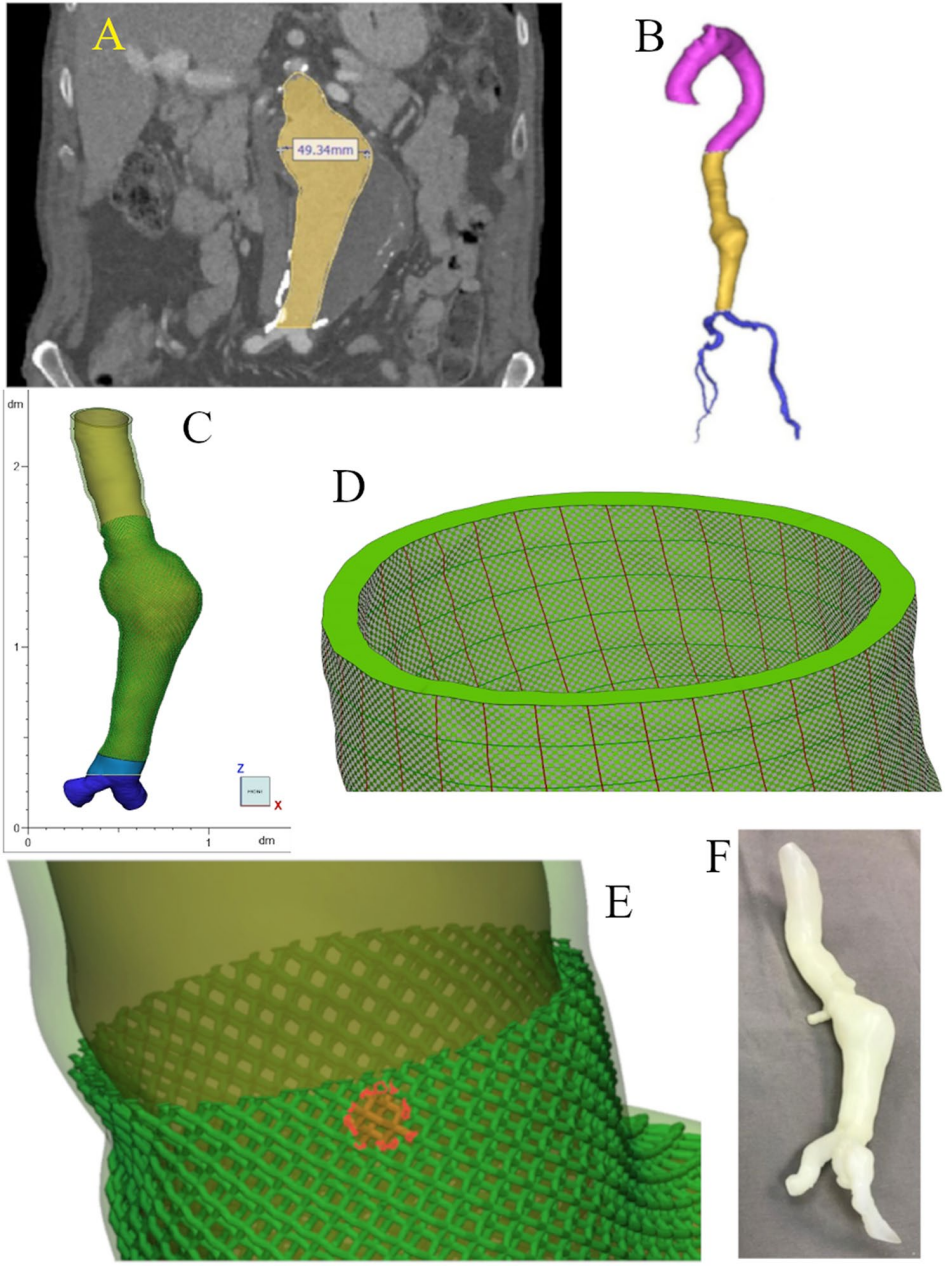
(A) Coronal view of the CT image showing the region of the aorta (in yellow) and the maximum diameter of the aneurysm. (B) 3D model of the segmentation, divided in the ascendant and the thoracic aorta (pink), abdominal aorta (yellow), and iliac arteries (blue) sections. (C) Final model with the LR in the aortic wall. (D) UV maps created to align the unit cell to the vessel wall. (E) Detail of the final model with and without the LR, including one marked unit cell. (F) Photograph of the printed model of the abdominal aortic aneurysm withn no reinforcement.
